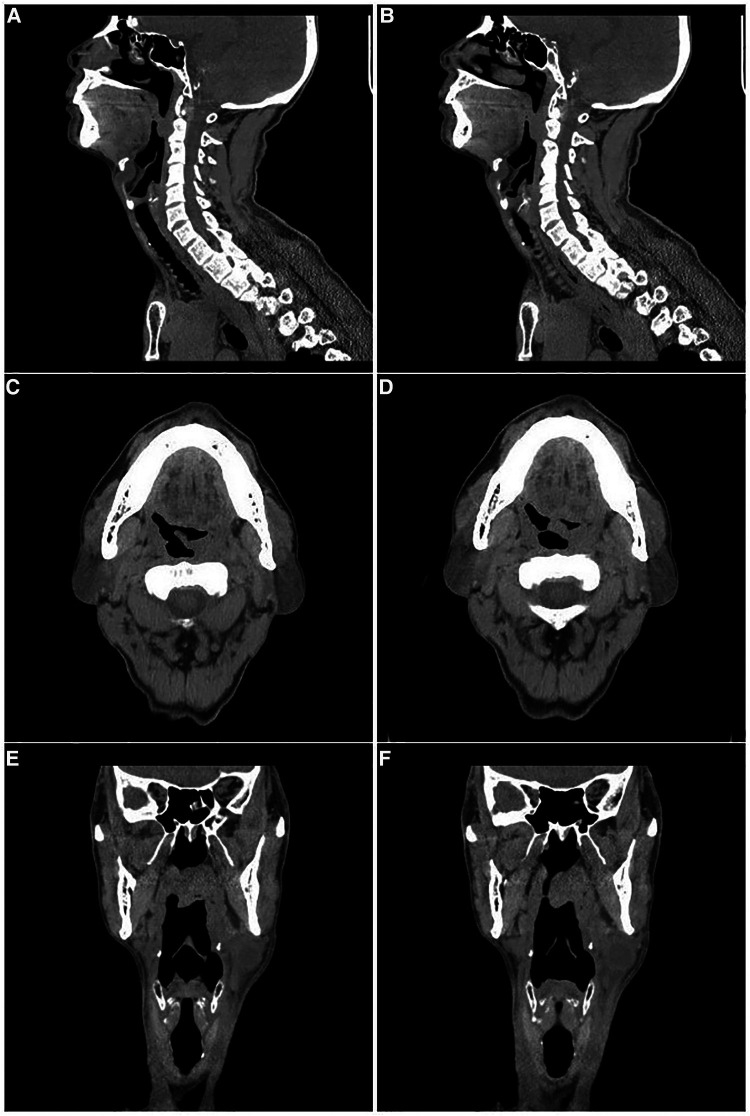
The CT images of the left soft palate. (A)–(B): Sagittal view. (C)–(D): Transverse view. (E)–(F): Coronal view.
